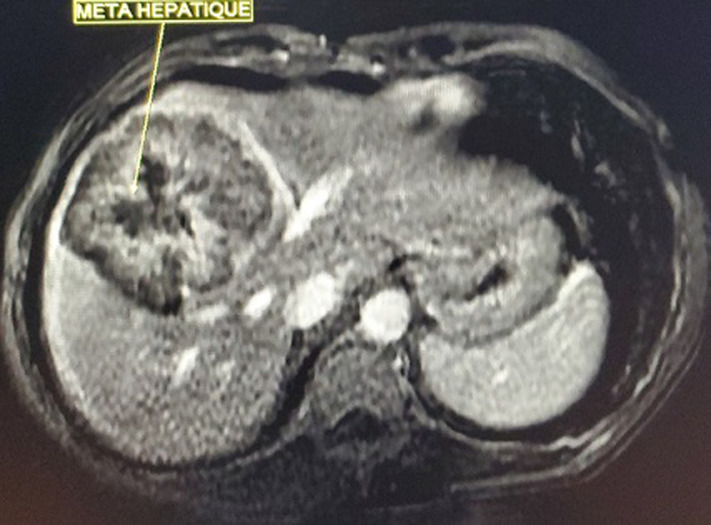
preoperative magnetic resonance imaging of the liver showing hepatic lesions with central necrosis
